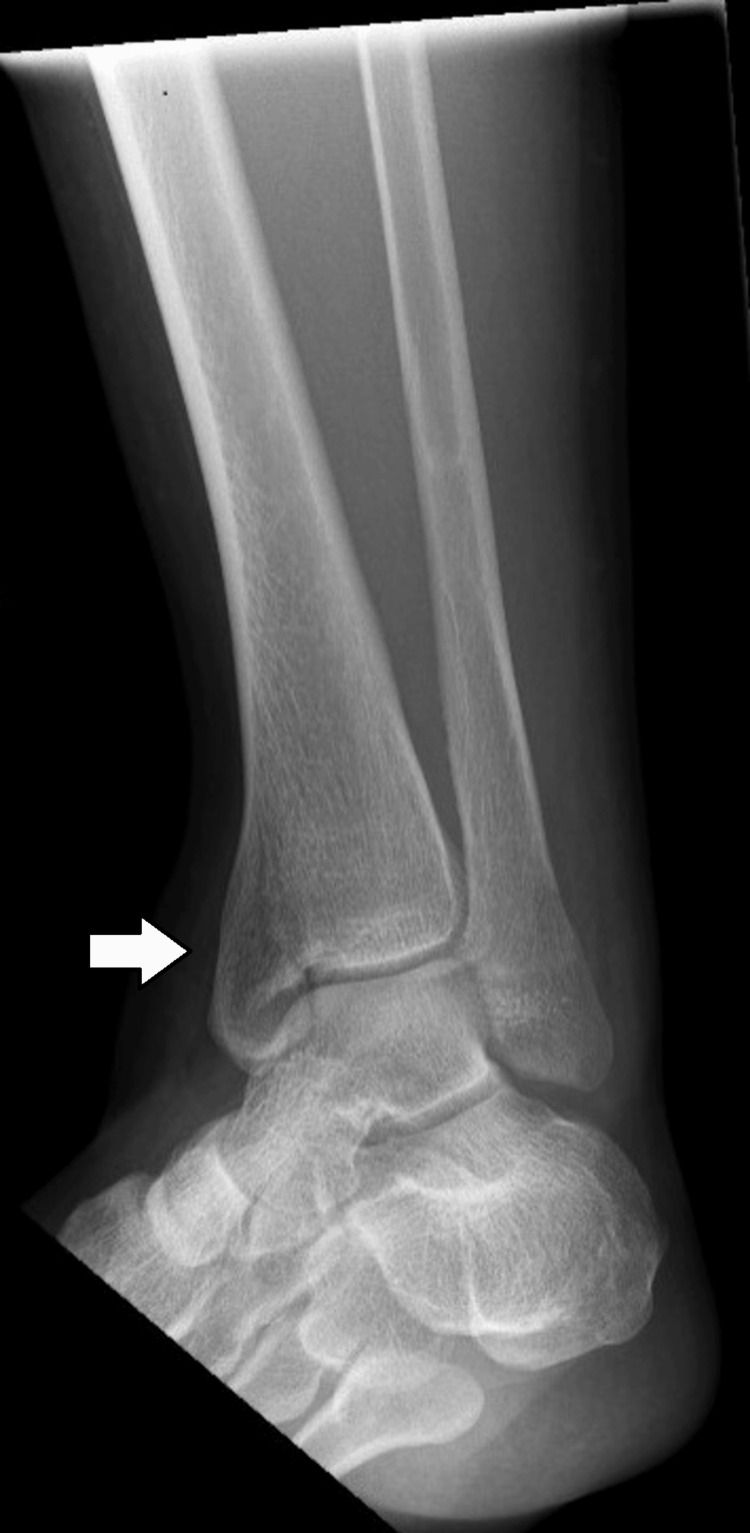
Rotated PA view of the left ankle on admission to the emergency department. The white arrow on the radiograph indicates the medial malleolar fracture. PA: posteroanterior
